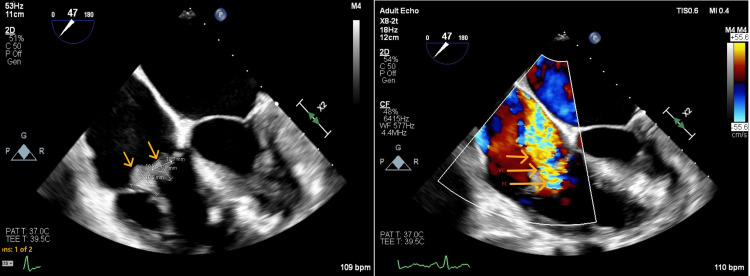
Transesophageal echocardiogram (tricuspid valve view) Arrows indicate tricuspid valve leaflet vegetations (left panel). Arrows also highlight flow convergence (FC), vena contracta (VC), and jet expansion (JE) on color flow Doppler, demonstrating tricuspid regurgitation (right panel).
